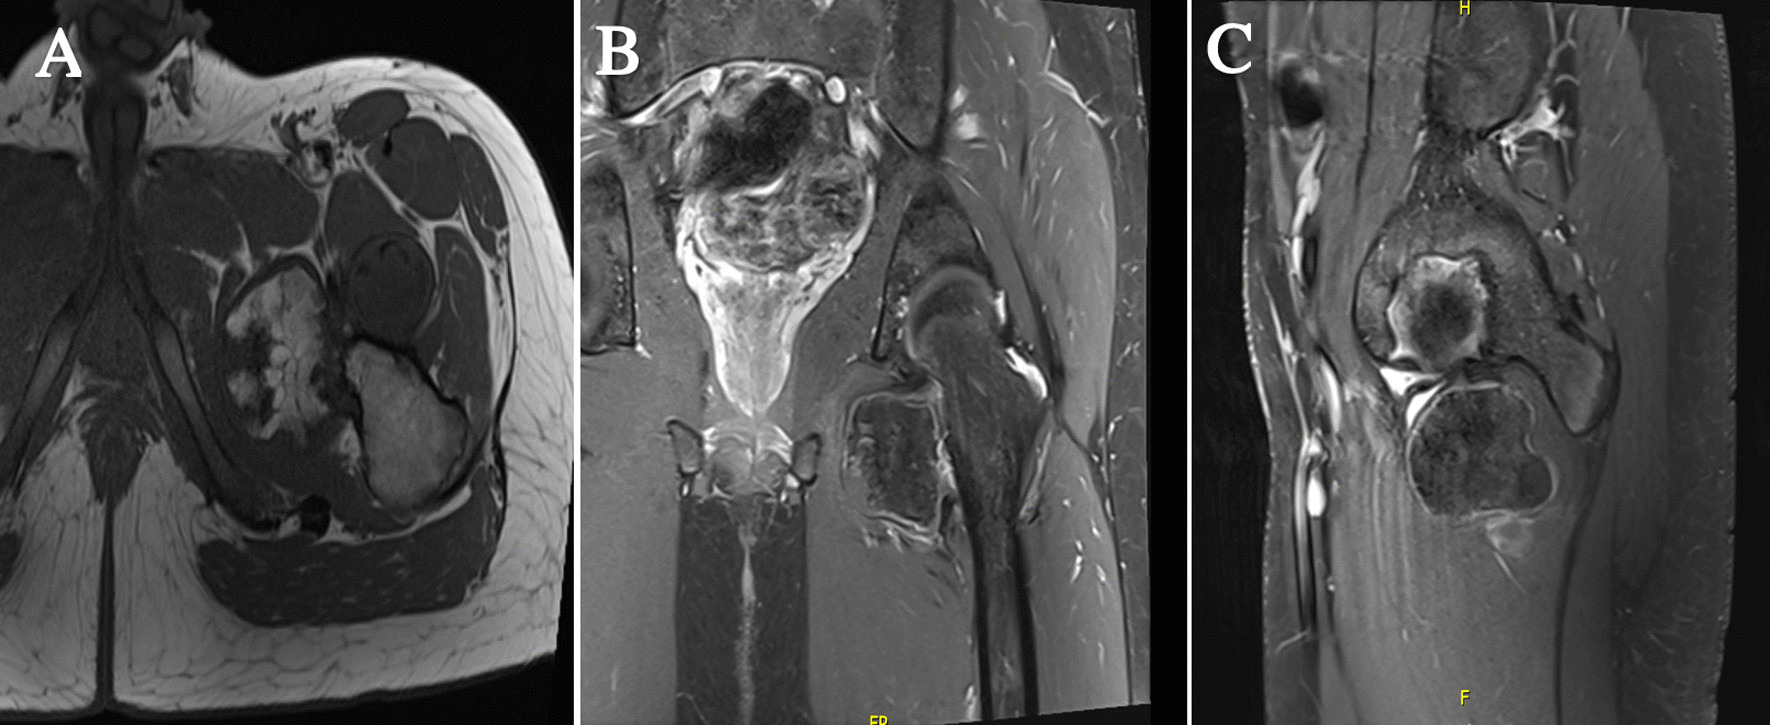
A Axial T1, B coronal T1, and C sagittal T1-weighted MRI scans of the left hip showing the inferomedial lesion with a cartilaginous rim and mature internal ossification
